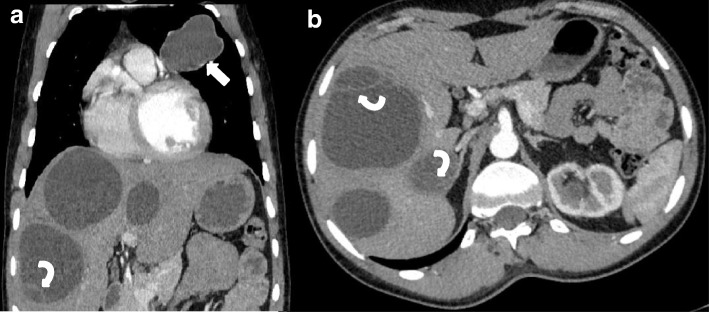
a-b: Contrast enhanced chest and upper abdominal CT coronal and axial sections: A well-defined, thin walled, low attenuating, cystic lesion with lobulated outlines in the anterior segment of the left upper lobe (arrow) and at least three cystic lesion in the liver one of them showing internal membrane (Curved arrows)
